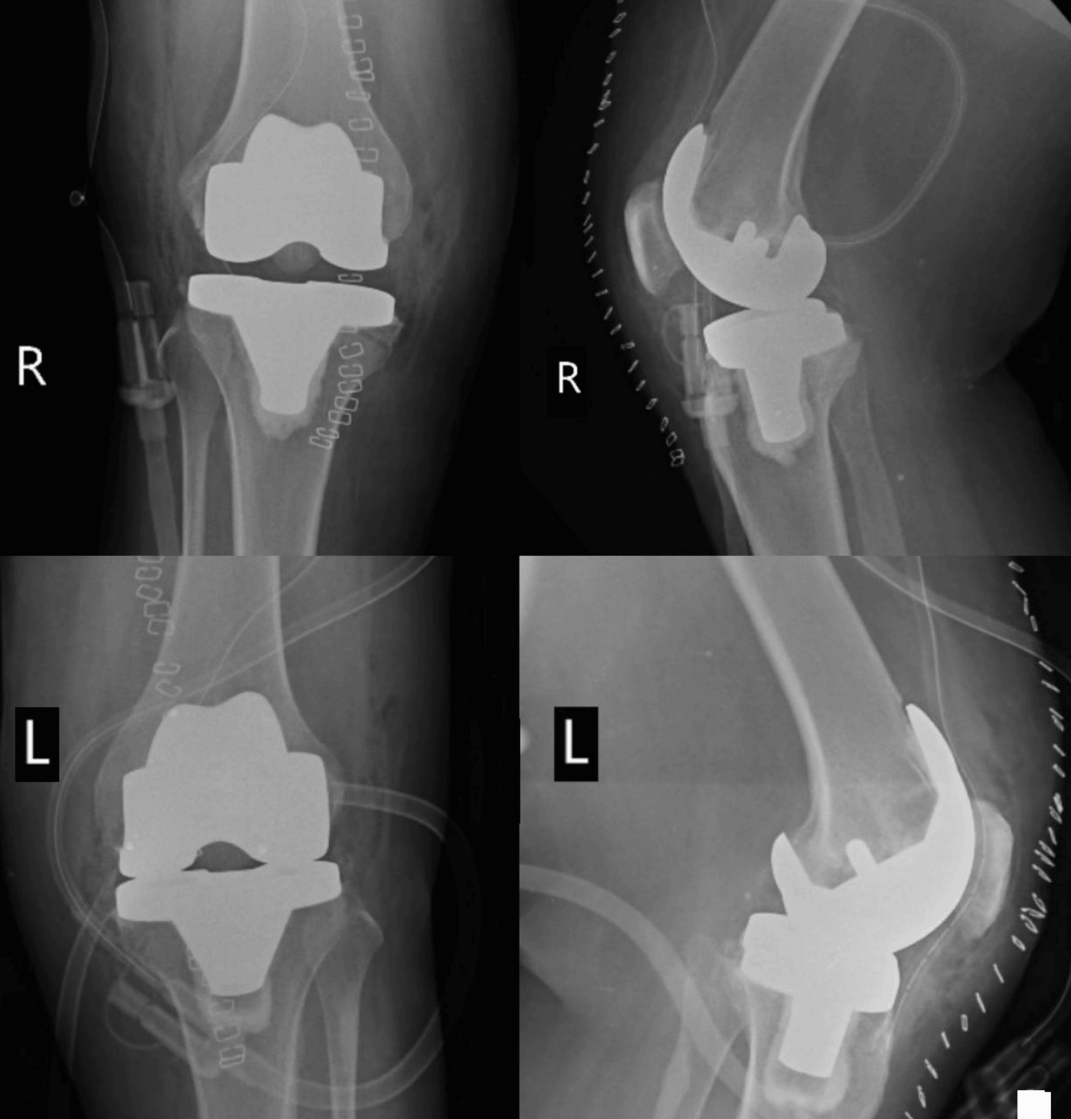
Postoperative X-ray of both knees showing implants
